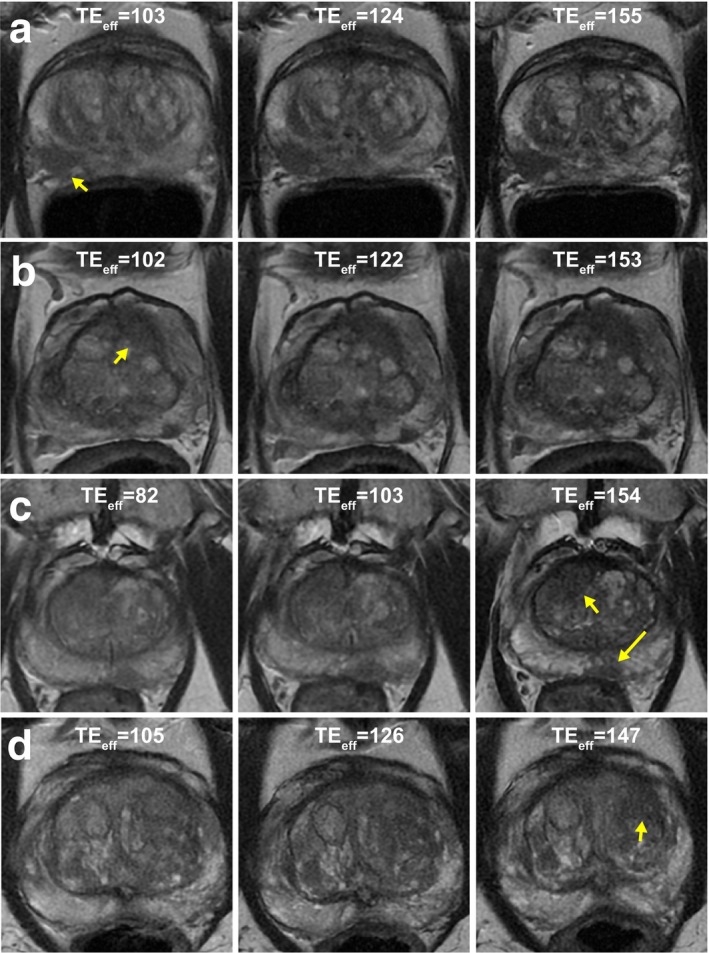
In vivo examples from four subjects with prostate cancer (PCa) imaged with different TE_eff_ values. For each subject shown, a biopsy was performed subsequent to the research MRI exam, and the Gleason Score (GS) is indicated. Lesions are identified with yellow arrows. (a) Peripheral zone (PZ) lesion, GS 4 + 3. (b) Transition zone (TZ) lesion, GS 3 + 4. (c) TZ lesion (short arrow), GS 3 + 4; PZ lesion (long arrow), GS 4 + 3. (d) TZ lesion, GS 3 + 3. In general, the visual contrast‐to‐background tissue ratio improves as TE_eff_ increases.
